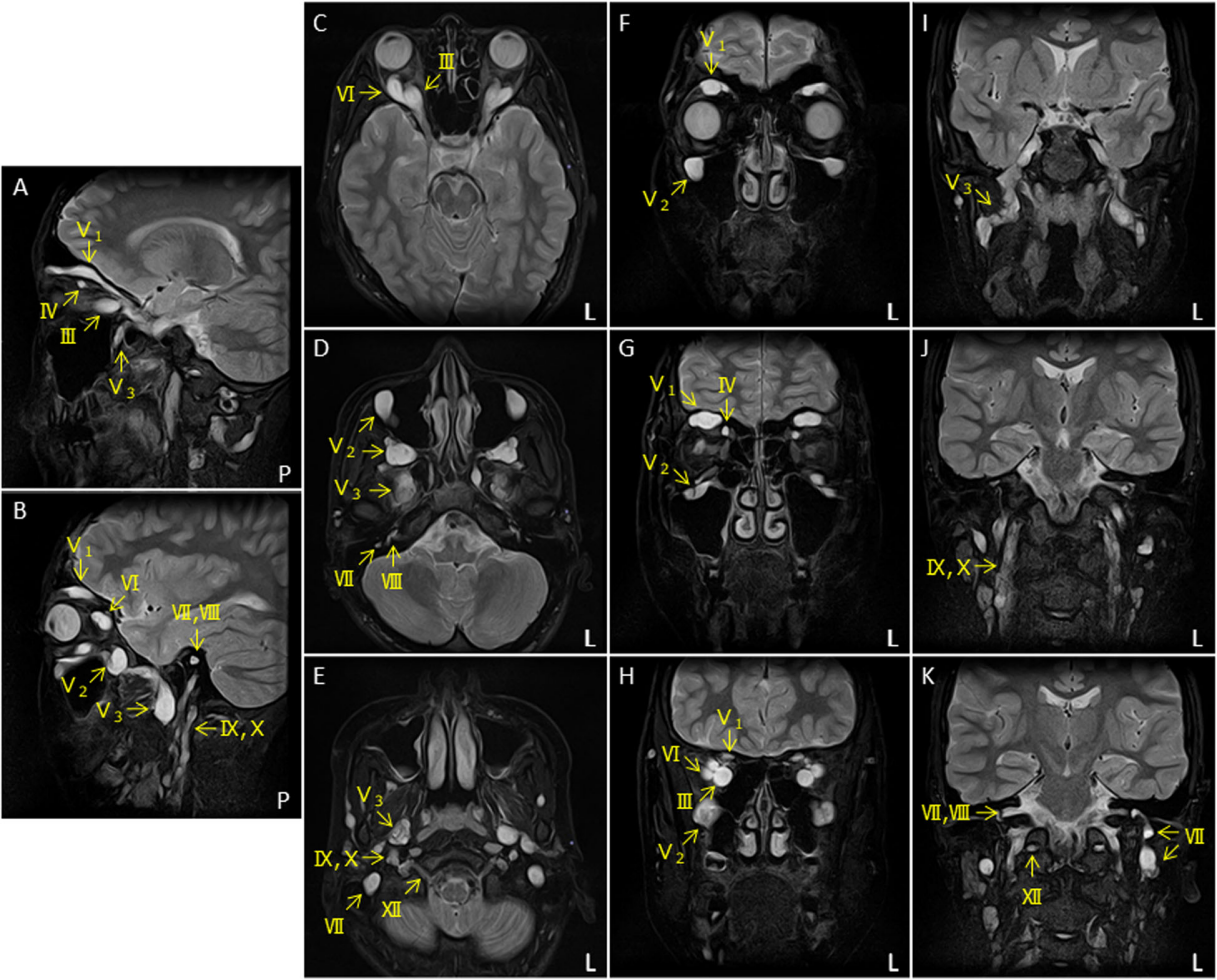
Magnetic resonance imaging of the cranial nerves. Sagittal (A-B), axial (C-E), and coronal (F-K) T2-weighted images reveal thickened cranial nerves except the olfactory and optic nerves. Abbreviations, III: oculomotor nerve, IV: trochlear nerve, V1: ophthalmic nerve, V2: maxillary nerve, V3: mandibular nerve, VI: abducens nerve, VII: facial nerve, VII: auditory nerve, IX: glossopharyngeal nerve, X: vagus nerve, XII: hypoglossal nerve, L: left, and P: Posterior
